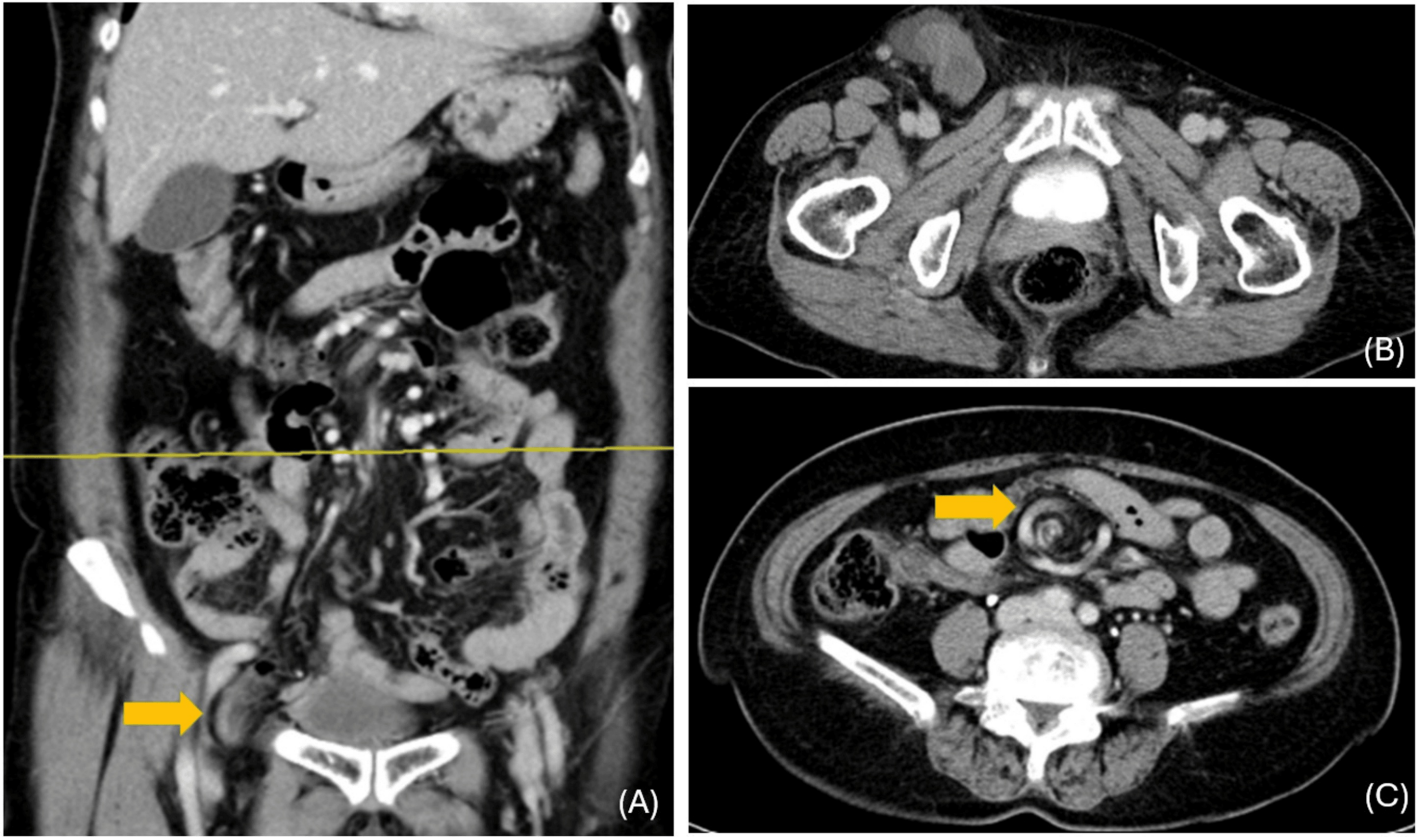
CT findings of an incarcerated femoral hernia (A) Coronal view demonstrates herniation of a small-bowel loop below the inguinal ligament into the right femoral canal (yellow arrow), confirming an incarcerated femoral hernia. (B) Axial pelvic CT at the level of the femoral canal shows a small bowel loop protruding medial to the right femoral vein, consistent with an incarcerated femoral hernia. (C) Axial view shows a mesenteric “whirl sign” (yellow arrow), consistent with volvulus of the small bowel in the setting of groin hernia incarceration.
